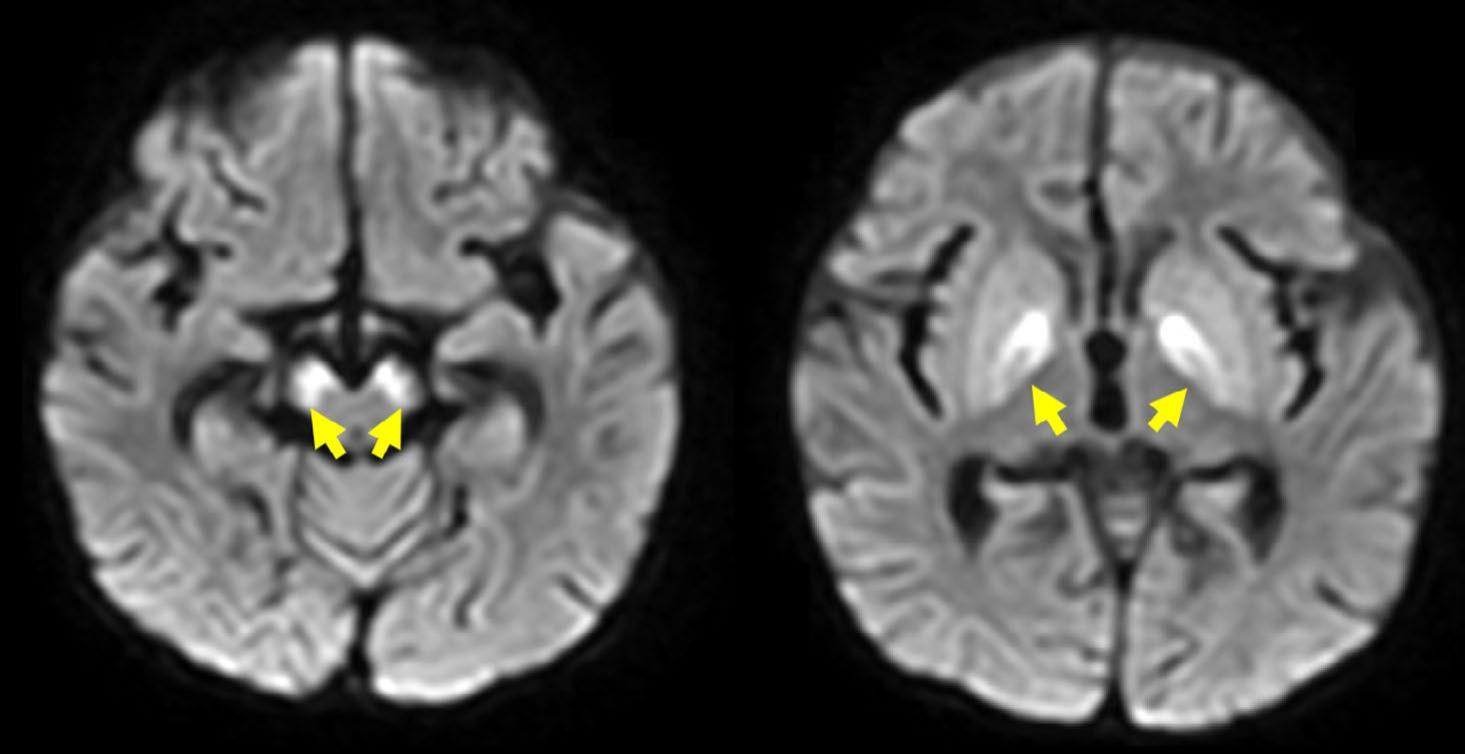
MRI on Day 7 after Admission. MRI showing abnormal signals in the bilateral globus pallidus and substantia nigra on diffusion‐weighted imaging (yellow arrows).
